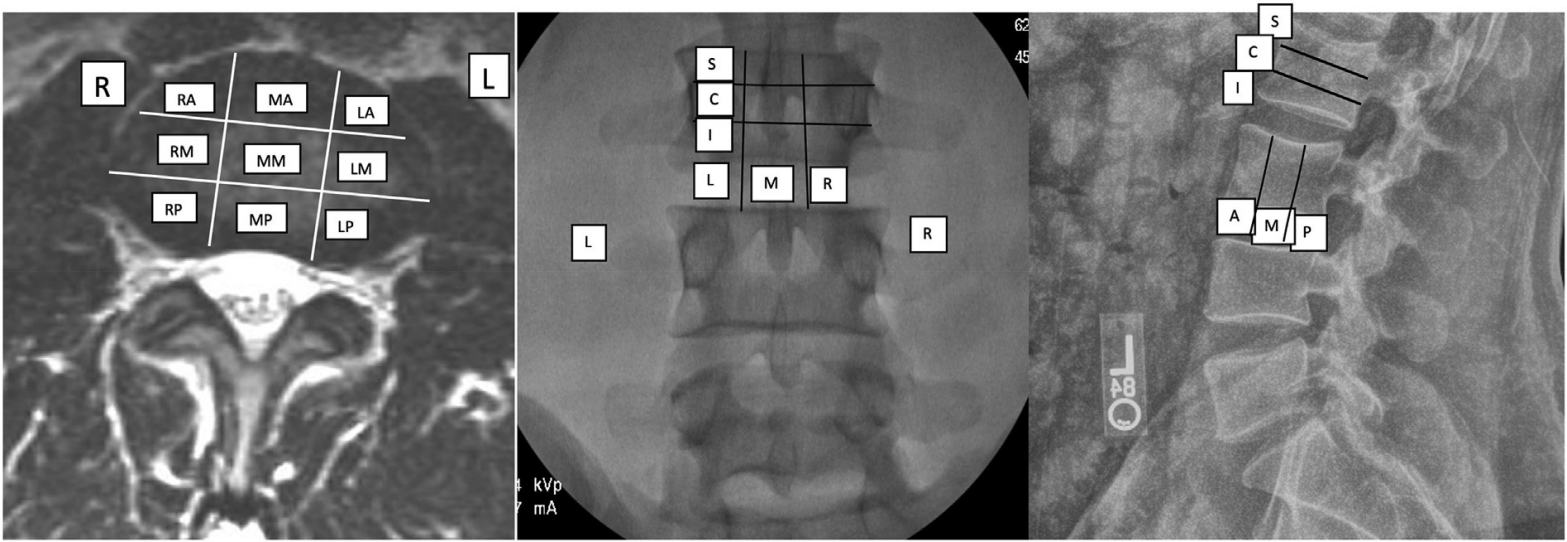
Zones for disc and vertebral body reporting, lumbar MRI, lumbar AP radiograph (right and left are flipped) and lumbar lateral radiograph. R – right, M −middle (both AP and lateral dimension), L-left, A-anterior, P – posterior, S- superior, C- central, I - inferior.
